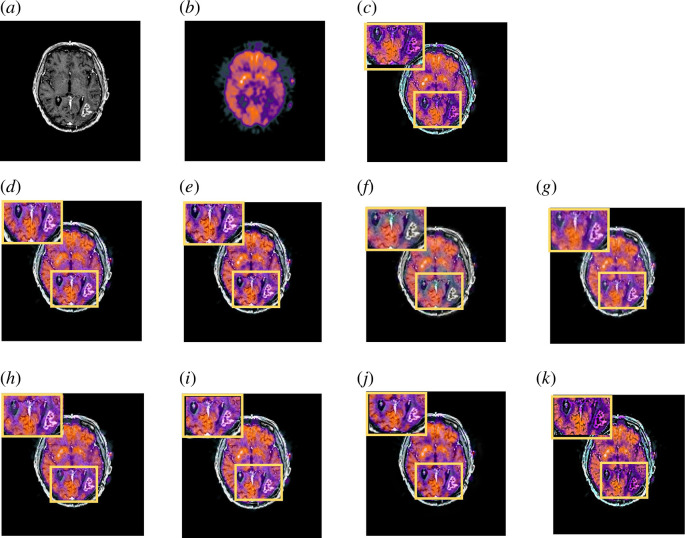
Comparison of fused image on the MRI-PET from Glioma image dataset. (*a*) original MRI image. (*b*) original PET image. (*c*) MLMG-PCNN [16]. (*d*) JBF [18]. (*e*) Local extreme map GF [39]. (*f*) LRD [7]. (*g*) ACOF [40]. (*h*) MDHU [41]. (*i*) FGF [42]. (*j*) Fusion based on phase congruency [43]. (*k*) Proposed.
